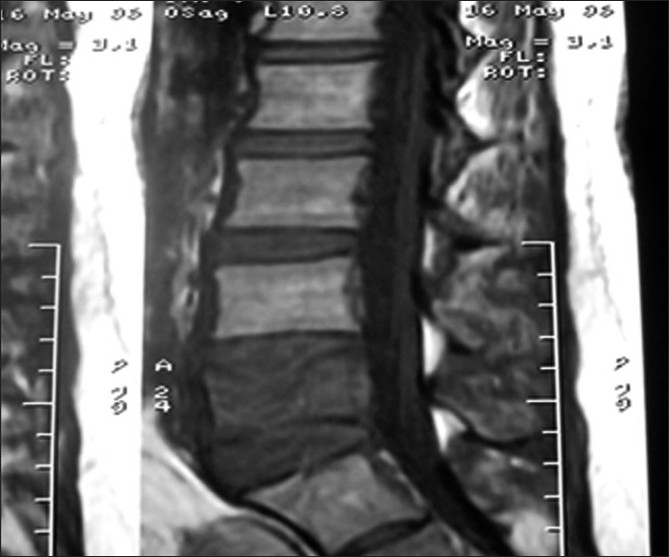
T1W MRI mid sagittal scan showing destruction of the L5 vertebra
